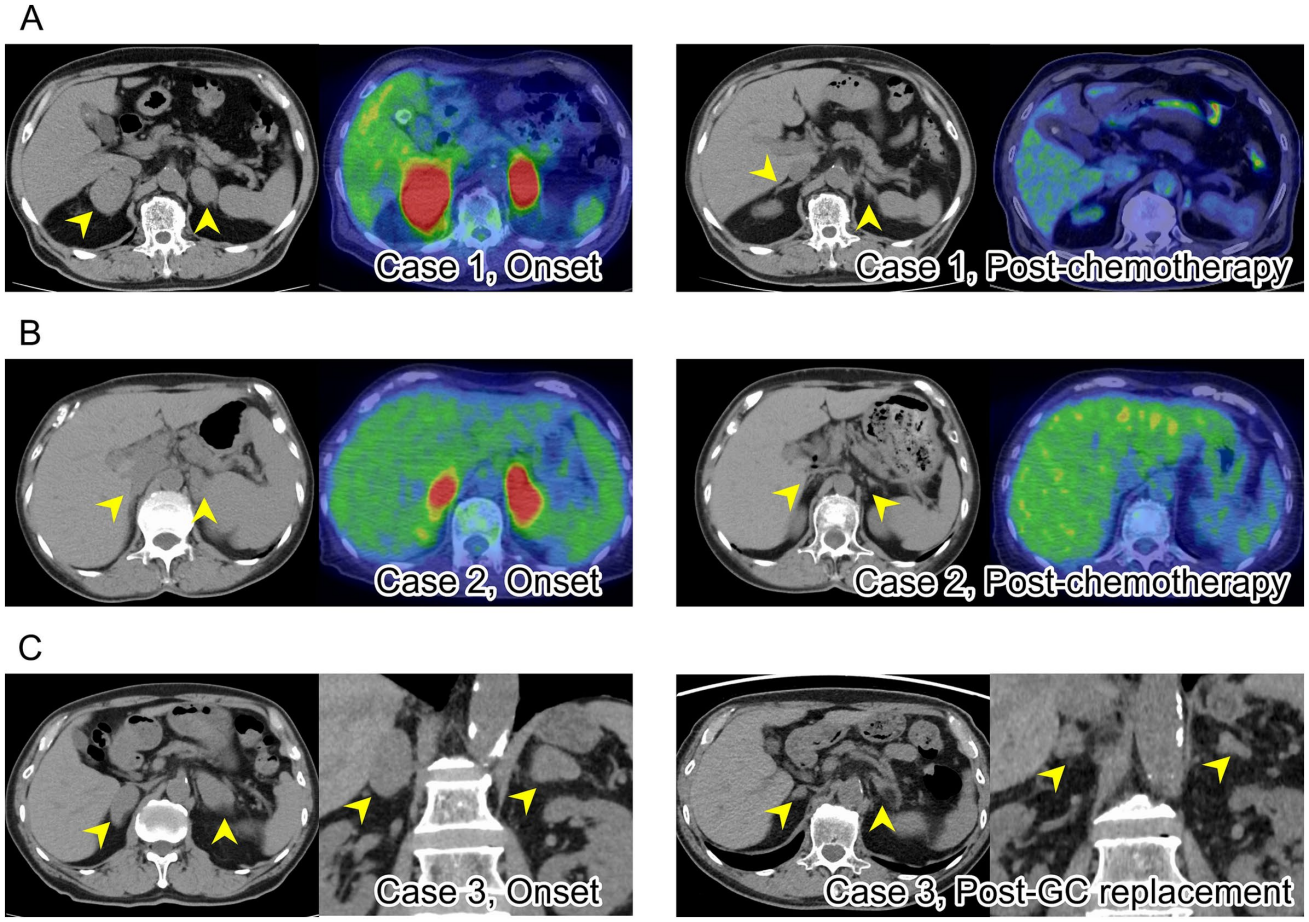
(A) Left panel: a plain computed tomography (CT) in case 1 showed bilateral adrenal masses (arrows); ^18^F-fluorodeoxyglucose positron emission tomography (FDG-PET)/CT showed abnormal tracer accumulation in both adrenal glands. Right panel: CT in case 1 after chemotherapy for lymphoma showed shrinkage of the masses (arrows) and decreased FDG accumulation. (B) Left panel: CT in case 2 showed bilateral adrenal masses (arrows); FDG accumulation was confirmed in both adrenal glands. Right panel: CT in case 2 after chemotherapy showed shrinkage of the masses (arrows) with normalized FDG accumulation. (C) Left panel: CT in case 3 showed bilateral adrenal masses (arrows). Right panel: CT in case 3 after corticosteroid replacement therapy showed shrinkage of the masses (arrows).
